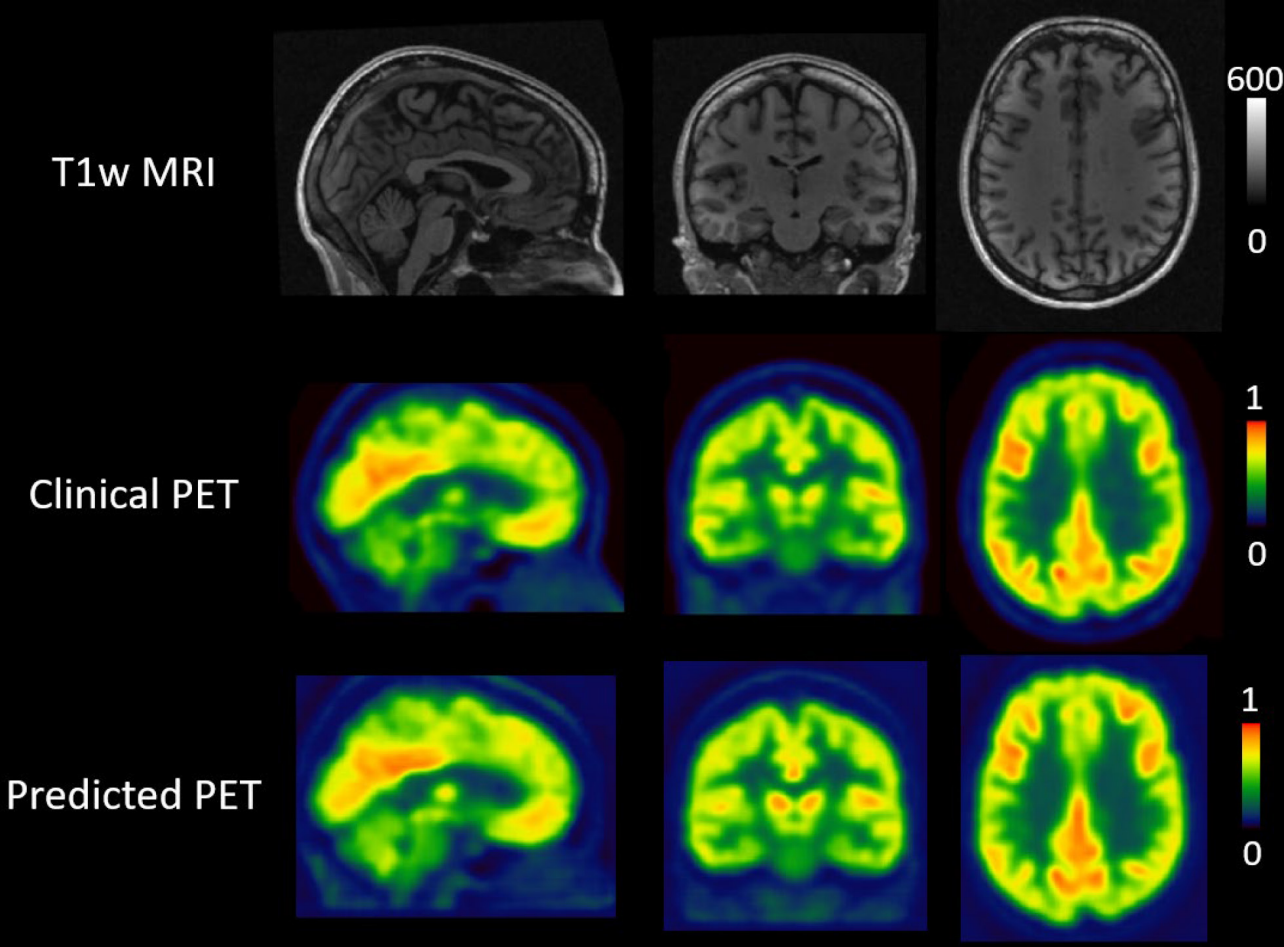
GAN results for one representative control subject. The first row shows the T1w MRI, the second row the clinical [^18^F]FDG PET, and the third row the predicted [^18^F]FDG PET, ie, the output from the generative adversarial network. From left to right, sagittal, coronal, and transverse slices are shown for each set.
